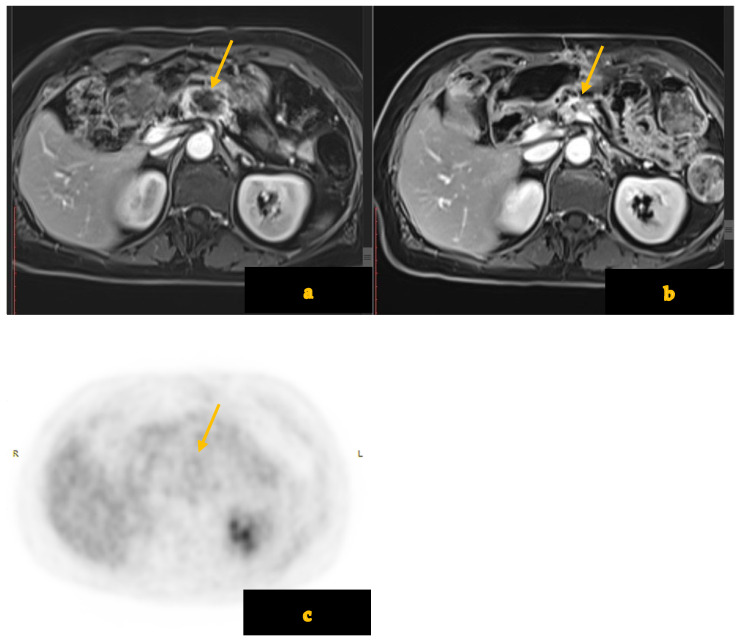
(a–c) Contrast-enhanced MRI shows a necrotic mass (arrow) in the pancreatic neck (a). After chemotherapy (b), there is a good response concerning the mass diameter and the vascular compression (arrow). FDG-PET (c) shows no uptake in the pancreas (arrow).
